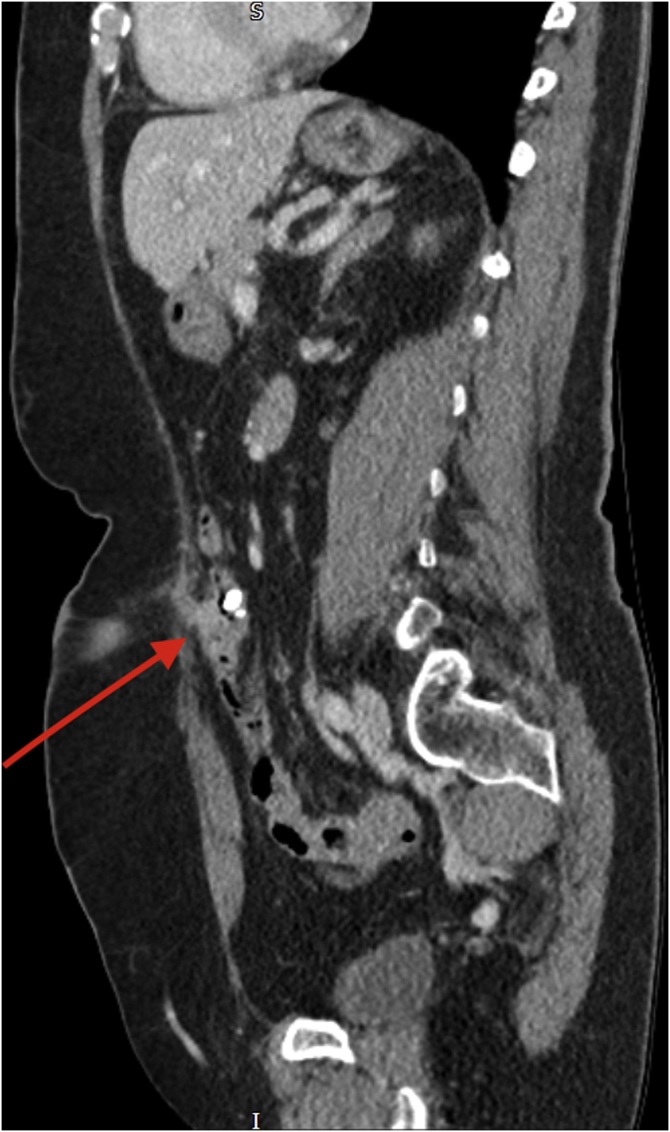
Contrast CT image of the ECF in the sagittal plane.
